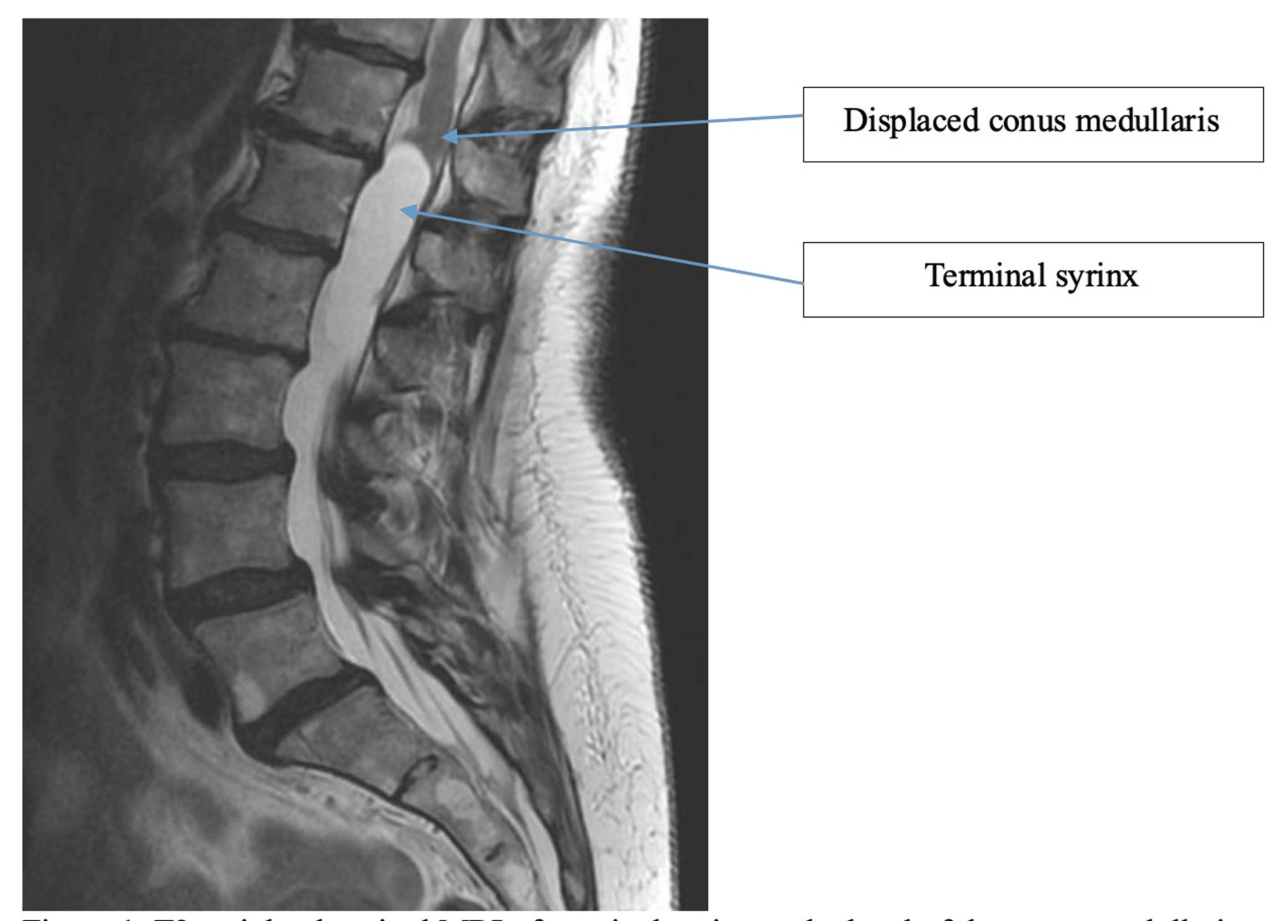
T2-weighted sagittal lumbar spine MRI of the terminal syrinx at the level of the conus medullaris The arrow points to the displaced conus medullaris and the terminal syrinx.
